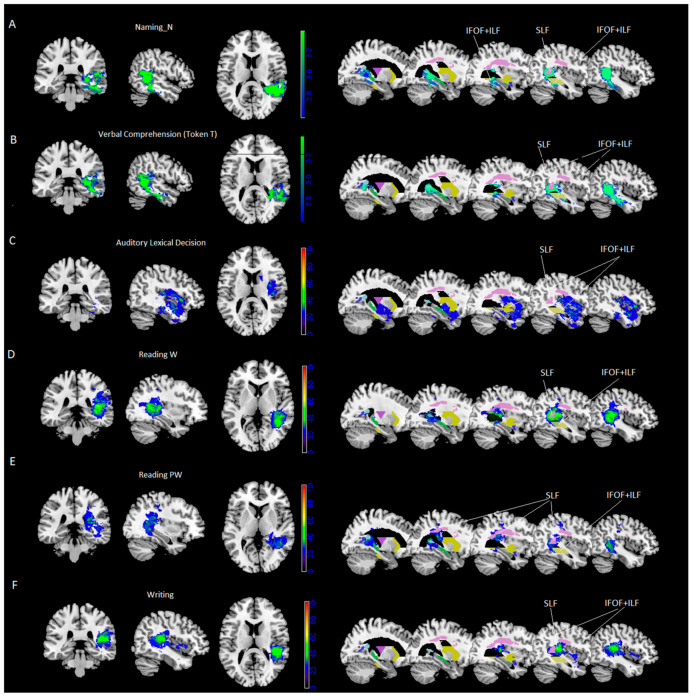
VLSM templates showing lesions significantly affecting performance on (A) noun naming and (B) token test. (*p* < 0.01, thresholds based on permutation method). Subtraction of lesion masks (C–F) of patients scoring pathologically from patients with normal performance (minimum 20% overlap) on the different tasks. MR images are displayed following radiological convention (left is right and vice versa). On the right side of the panel, results overlap with the JUH template of white matter pathways on sagittal slices. SLF = superior longitudinal fasciculus, IFOF = inferior fronto-occipital fasciculus; ILF = inferior longitudinal fasciculus belonging to sagittal stratum. N = nouns; W = words; PW = pseudowords; Token T = Token test.
